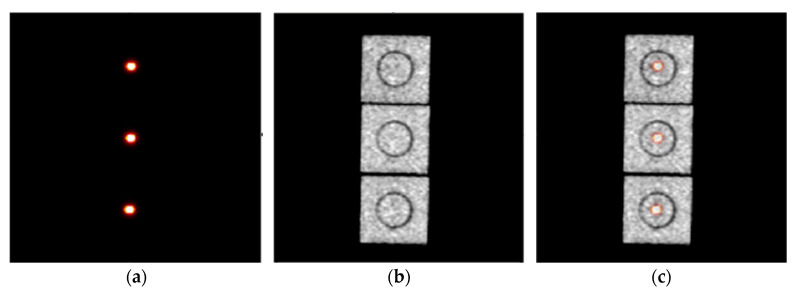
(a) PET and (b) CT images of the ^22^Na point source at three different FOV positions but viewed through the PET and CT sagittal slices, and (c) the corresponding registered PET and CT images of the point source before the PET coordinate system transformation. The difference between the PET and CT measured point source positions was used for PET and CT coordinate system alignment.
